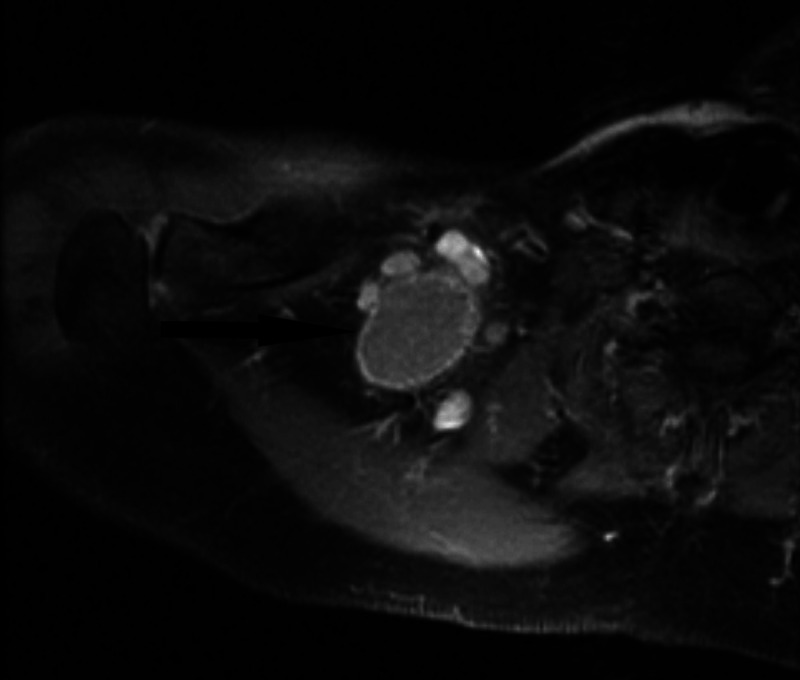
Right brachial plexus MRI T1 weighted showing the 3.3cm x 2.7cm mass.
